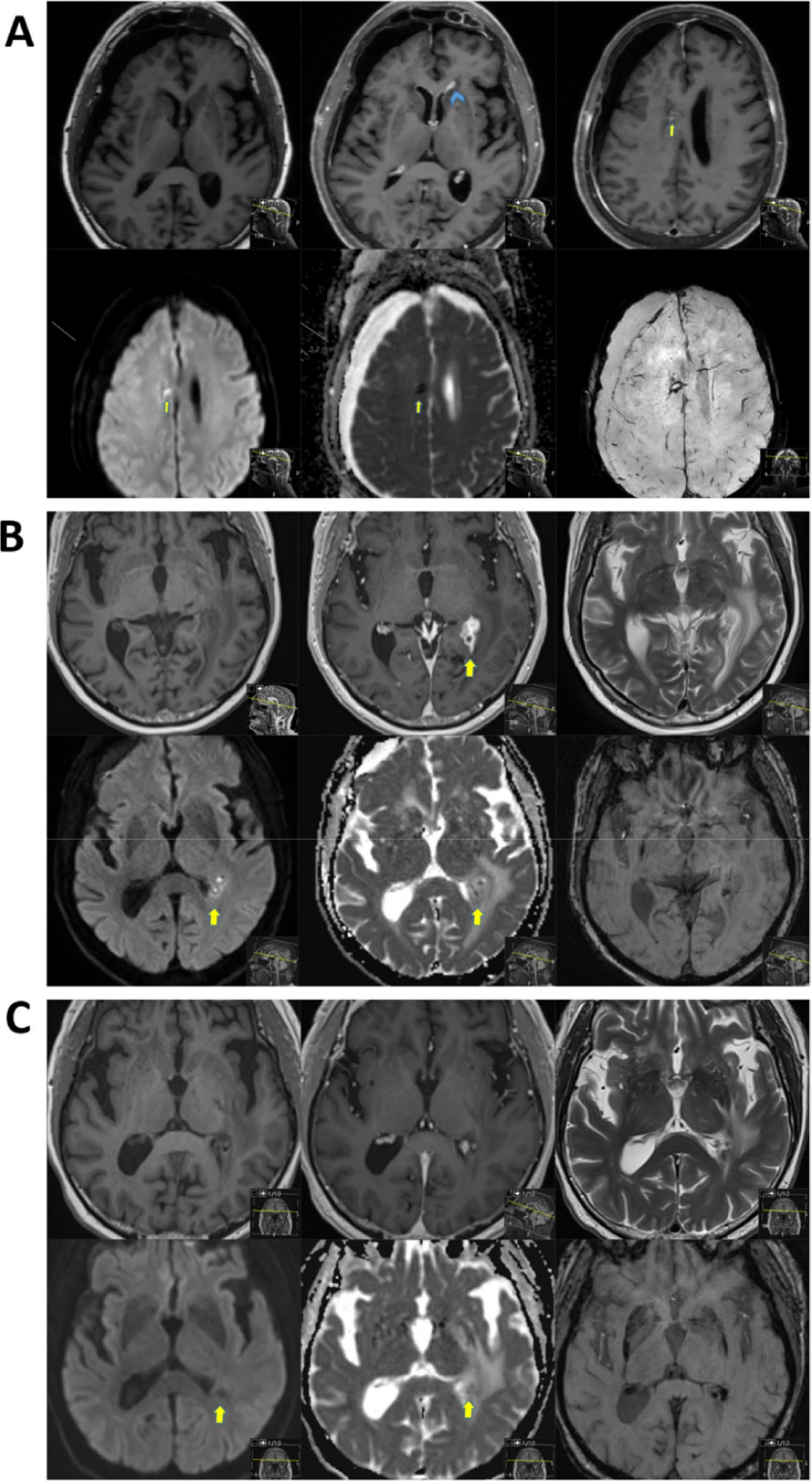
A) Axial native T1-w, CE T1-w (upper row), DWI, ADC, and SWI (lower row). Signs of meningitis with ventriculitis (blue arrowhead) as well as a suspected small periventricular abscess formation with signs of restricted diffusion (yellow arrow). Mass effect from a right-sided subdural hematoma, otherwise no obvious signs of increased intracranial pressure. B) Axial native T1-w, CE T1-w (upper row), DWI, ADC, and SWI (lower row). Clustered small intracranial abscesses near the left trigone with obstruction of the left temporal horn and ventriculitis (yellow arrow). The previously described right-sided subdural hematoma appears now subdivided into multiple compartments, likely with small portions of empyema (not shown in the image). Mild hydrocephalus is seen. C) Axial native T1-w, CE T1-w (upper row), DWI, ADC, and SWI (lower row). Regression of ventriculitis and abscesses is observed. Imaging shows no longer signs of restricted diffusion (yellow arrow), indicating an absence of active infection.
